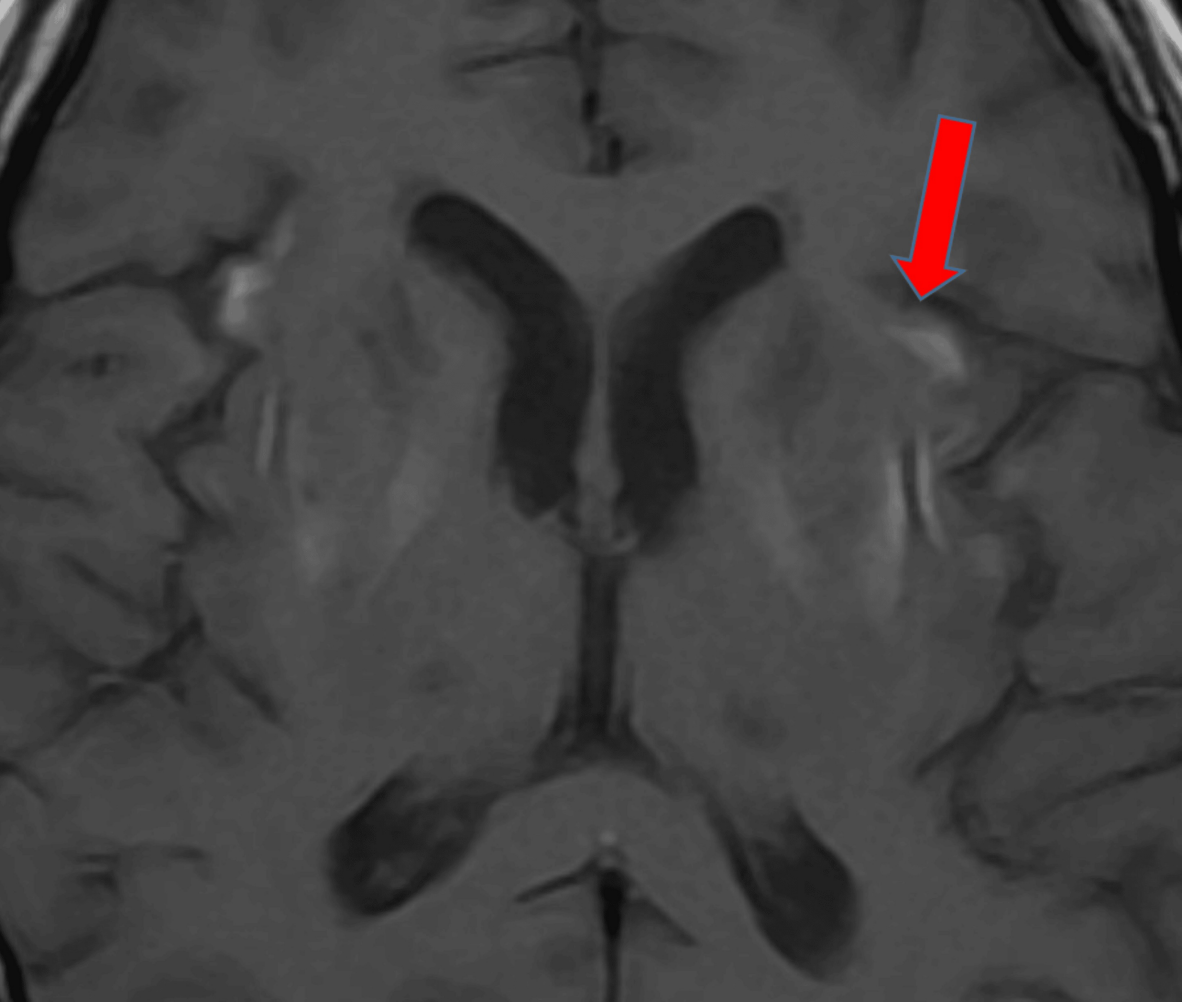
T1 Axial MRI Sequence Showing Hypointense Signal With Patchy Areas of Hyperintensity (Red Arrow), Suggestive of Hemorrhagic Transformation. MRI: magnetic resonance imaging
